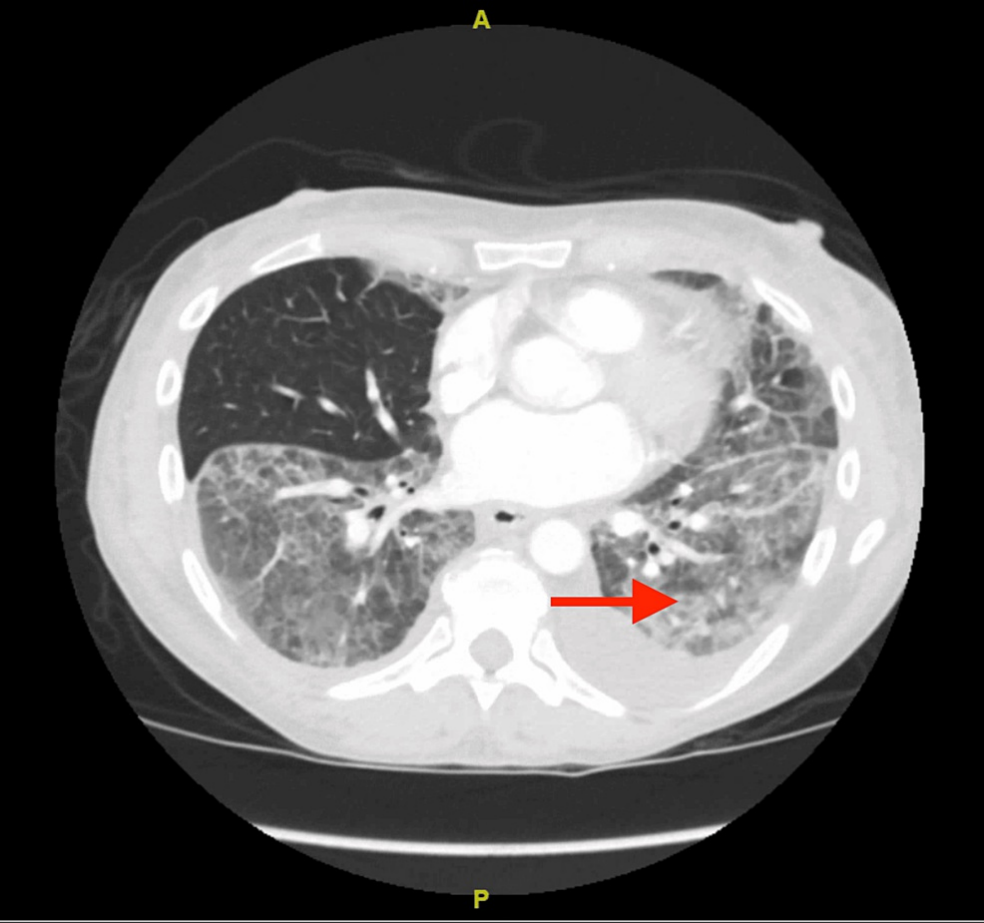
CT angiography of the chest showing bilateral ground glass opacities (red arrow) with sparing of the middle lobe.
